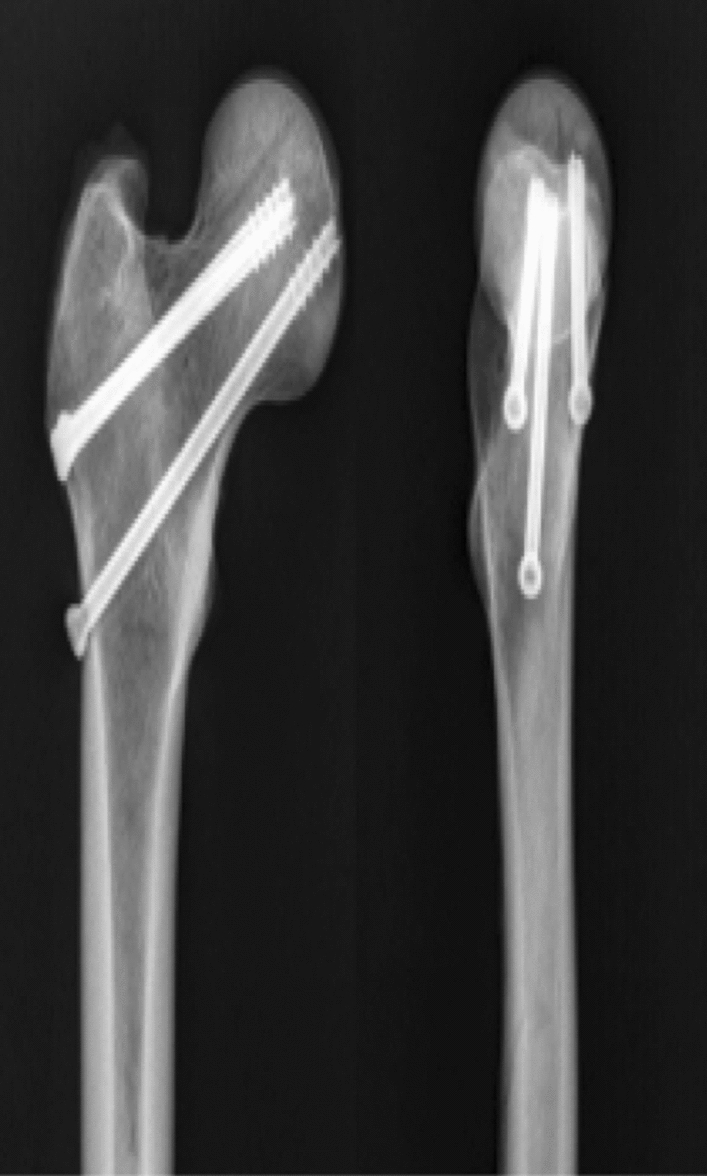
Three cannulated compression screws (3CCS) (*Note*: Positive and lateral X-ray film after internal fixation).
